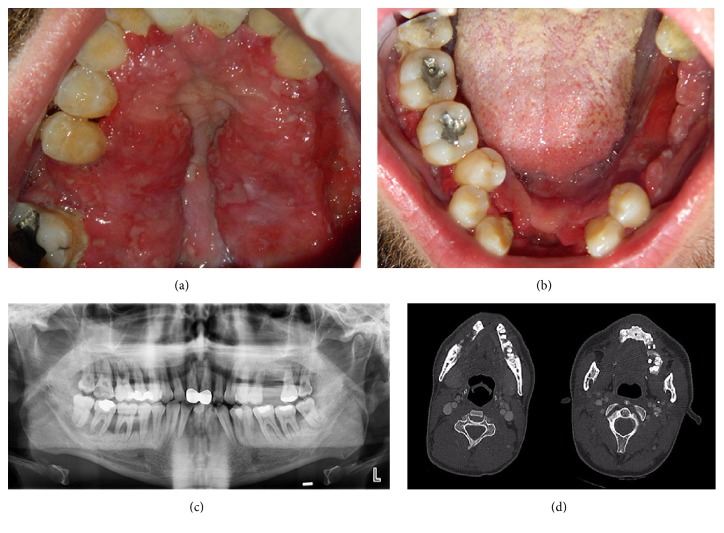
(a) Intraoral view of the patient after extraction of teeth with severe mobility, prior to initiation of chemotherapy. Extensive gingival lesions are observed on the palatal surface of the maxilla. (b) Intraoral view of the patient after extraction of teeth with severe mobility, prior to initiation of chemotherapy. Diffuse, erythematous, and ulcerated gingival hyperplasia is observed in the mandible. (c) Panoramic radiograph of the patient at initial presentation. Generalized alveolar bone loss is observed. (d) Axial sections of the computed tomography scan obtained at initial presentation. Diffuse involvement of both maxilla and the mandible is observed.
